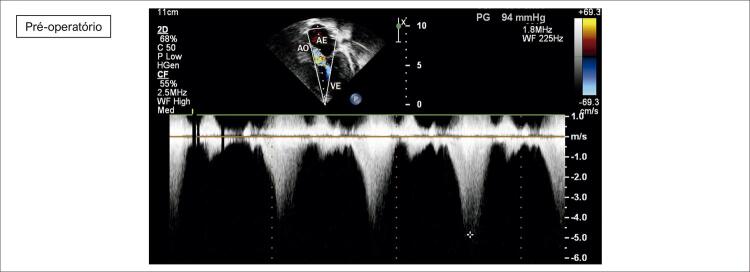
Corte apical cinco câmaras, evidenciando obstrução ao fluxo em via de saída de ventrículo ao color Doppler. Gradiente máximo obtido em via de saída ventricular = 94 mmHg. AO: aorta; AE: átrio esquerdo; VE: ventrículo esquerdo.
